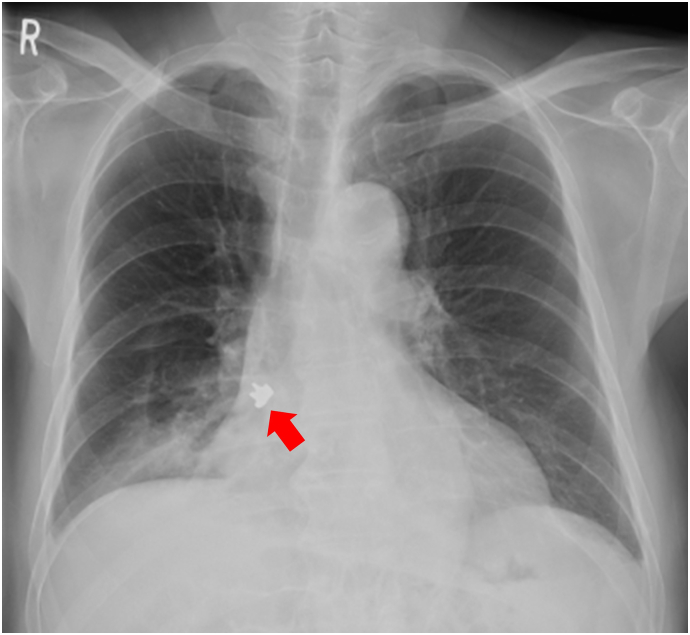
Chest roentgenography revealing a metallic foreign body (red arrow) in the right bronchus. (For interpretation of the references to colour in this figure legend, the reader is referred to the web version of this article.) (For interpretation of the references to colour in this figure legend, the reader is referred to the web version of this article.)
